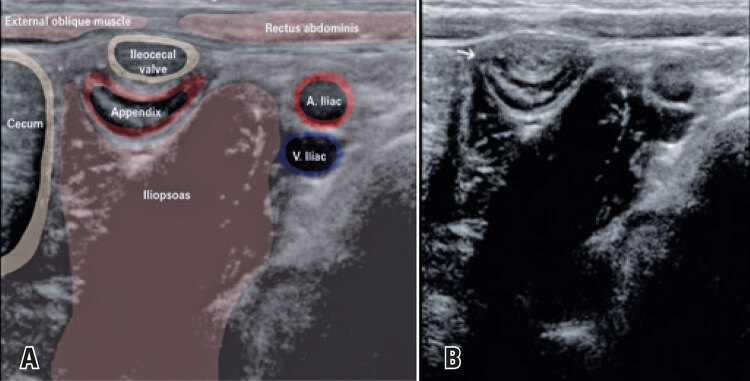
Sonographic images of abdominal structures. (A) Normal cecal appendix in the right iliac fossa (longitudinal section); (B) Typical blind end of this bowel- white arrow
